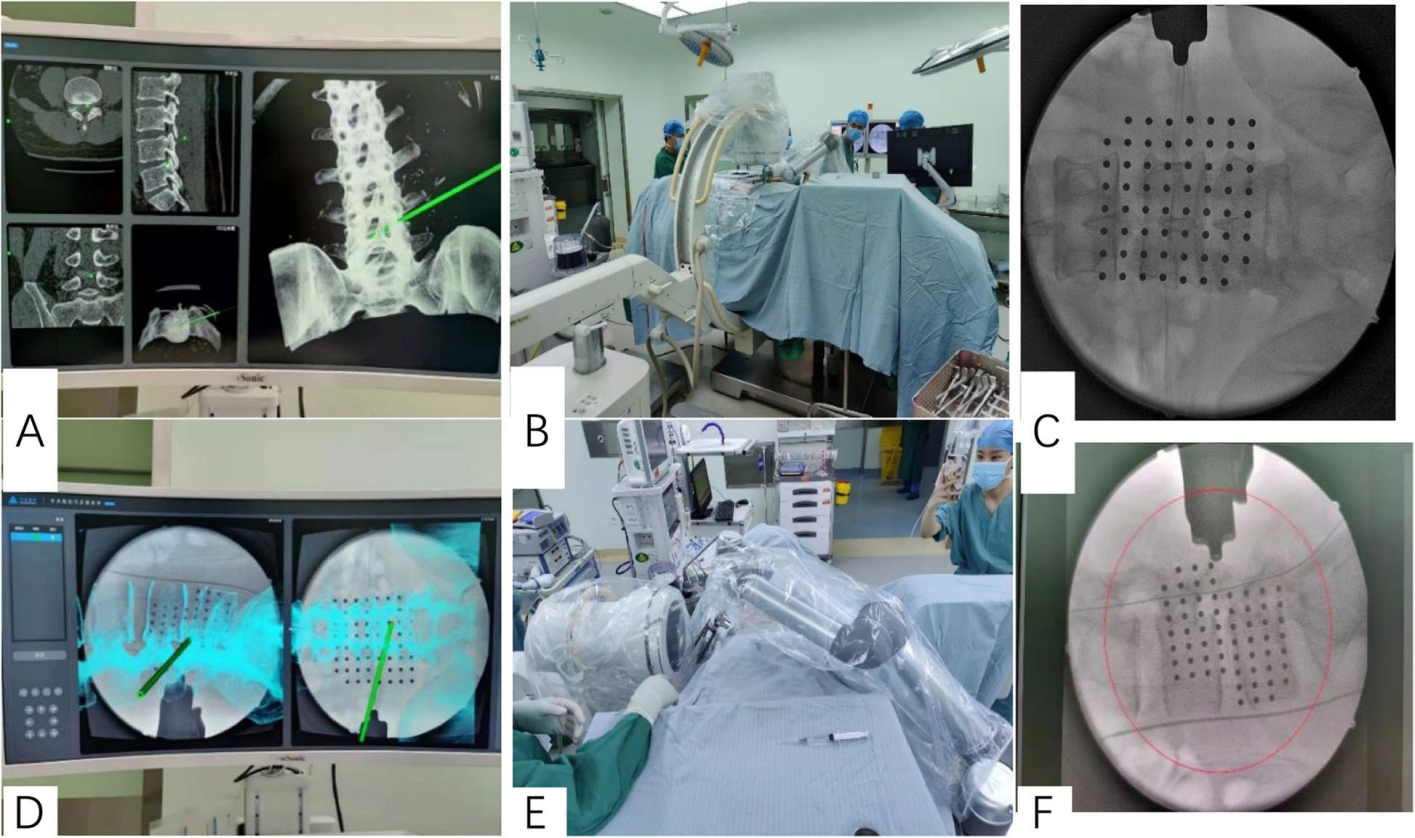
Fluoroscopic images are matched with CT data for the planning puncture trajectory. A The robotic system plans the puncture trajectory according to CT scan data is inputed into the working station. B, C, E, F) Anteroposterior and lateral fluoroscopic images are obtained while the robotic arm holds the reference frame. D)The fluoroscopic images are matched with the planning puncture trajectory based on CT data
